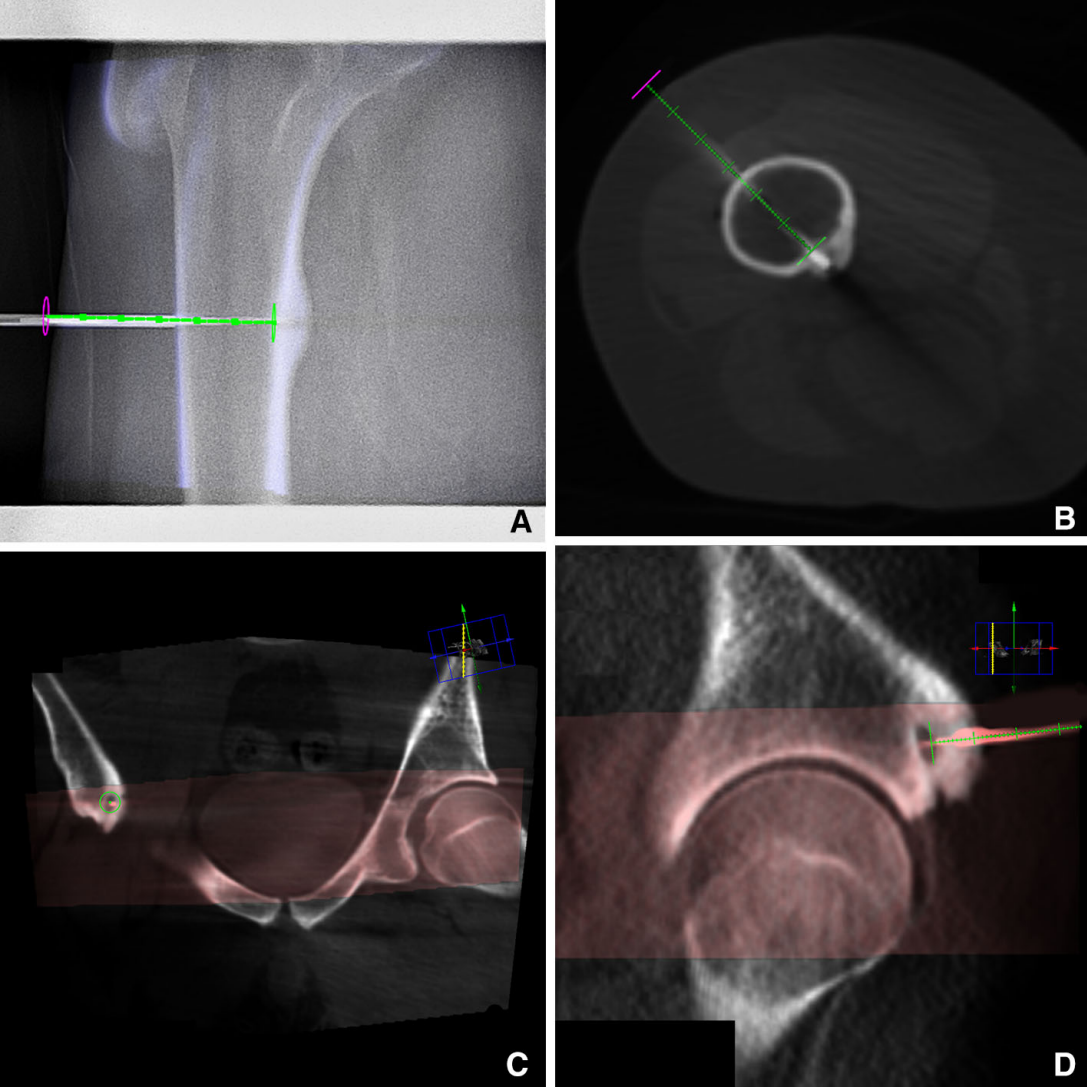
CBCT images of 2 patients with osteoid osteoma. A shows the overlay during fluoroscopy guidance in the progress view. B shows a CBCT scan of the same patient, with the RF electrode along the planned needle path (*green*). In C and D, the CBCT scan is fused with a previous CT scan, the CT scan is visualized in *gray* and the CBCT scan in *red*. In C, the entry *point view* is visualized and D shows the *progress view*
